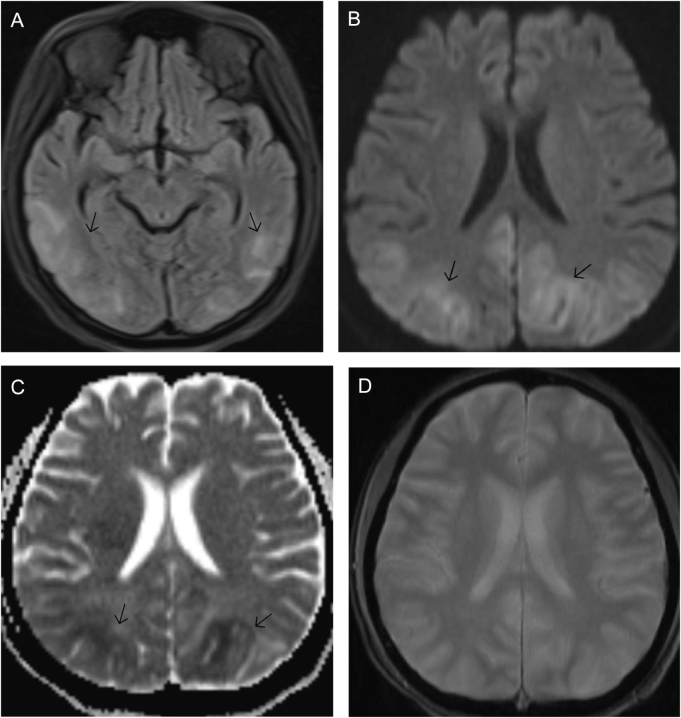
(A) Axial T2-FLAIR MRI of the brain at the level of the basal ganglia and temporal lobes demonstrates bilateral cortical and subcortical hyperintensities involving the posterior temporal and occipital regions (arrows), consistent with edematous changes. (B) Axial diffusion-weighted imaging (DWI) at the level of the posterior horns of the lateral ventricles shows bilateral hyperintense signal in the occipital lobes (arrows), indicating restricted diffusion. (C) Corresponding axial apparent diffusion coefficient (ADC) map at the same level demonstrates low ADC signal in the bilateral occipital regions (arrows), confirming true diffusion restriction. (D) Axial gradient echo (GRE) image at the corresponding ventricular level shows no blooming artefact.
